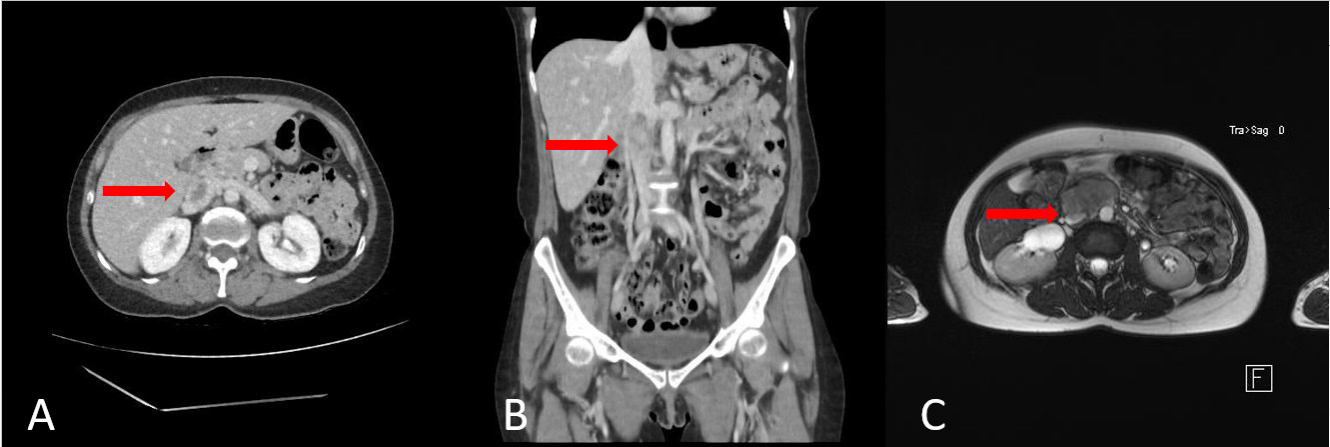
(A) and (B) Computed tomography angiography of the abdomen and pelvis in axial and coronal views, respectively; (C) Axial magnetic resonance in T2. Red arrows indicate the inferior vena cava leiomyosarcoma.
